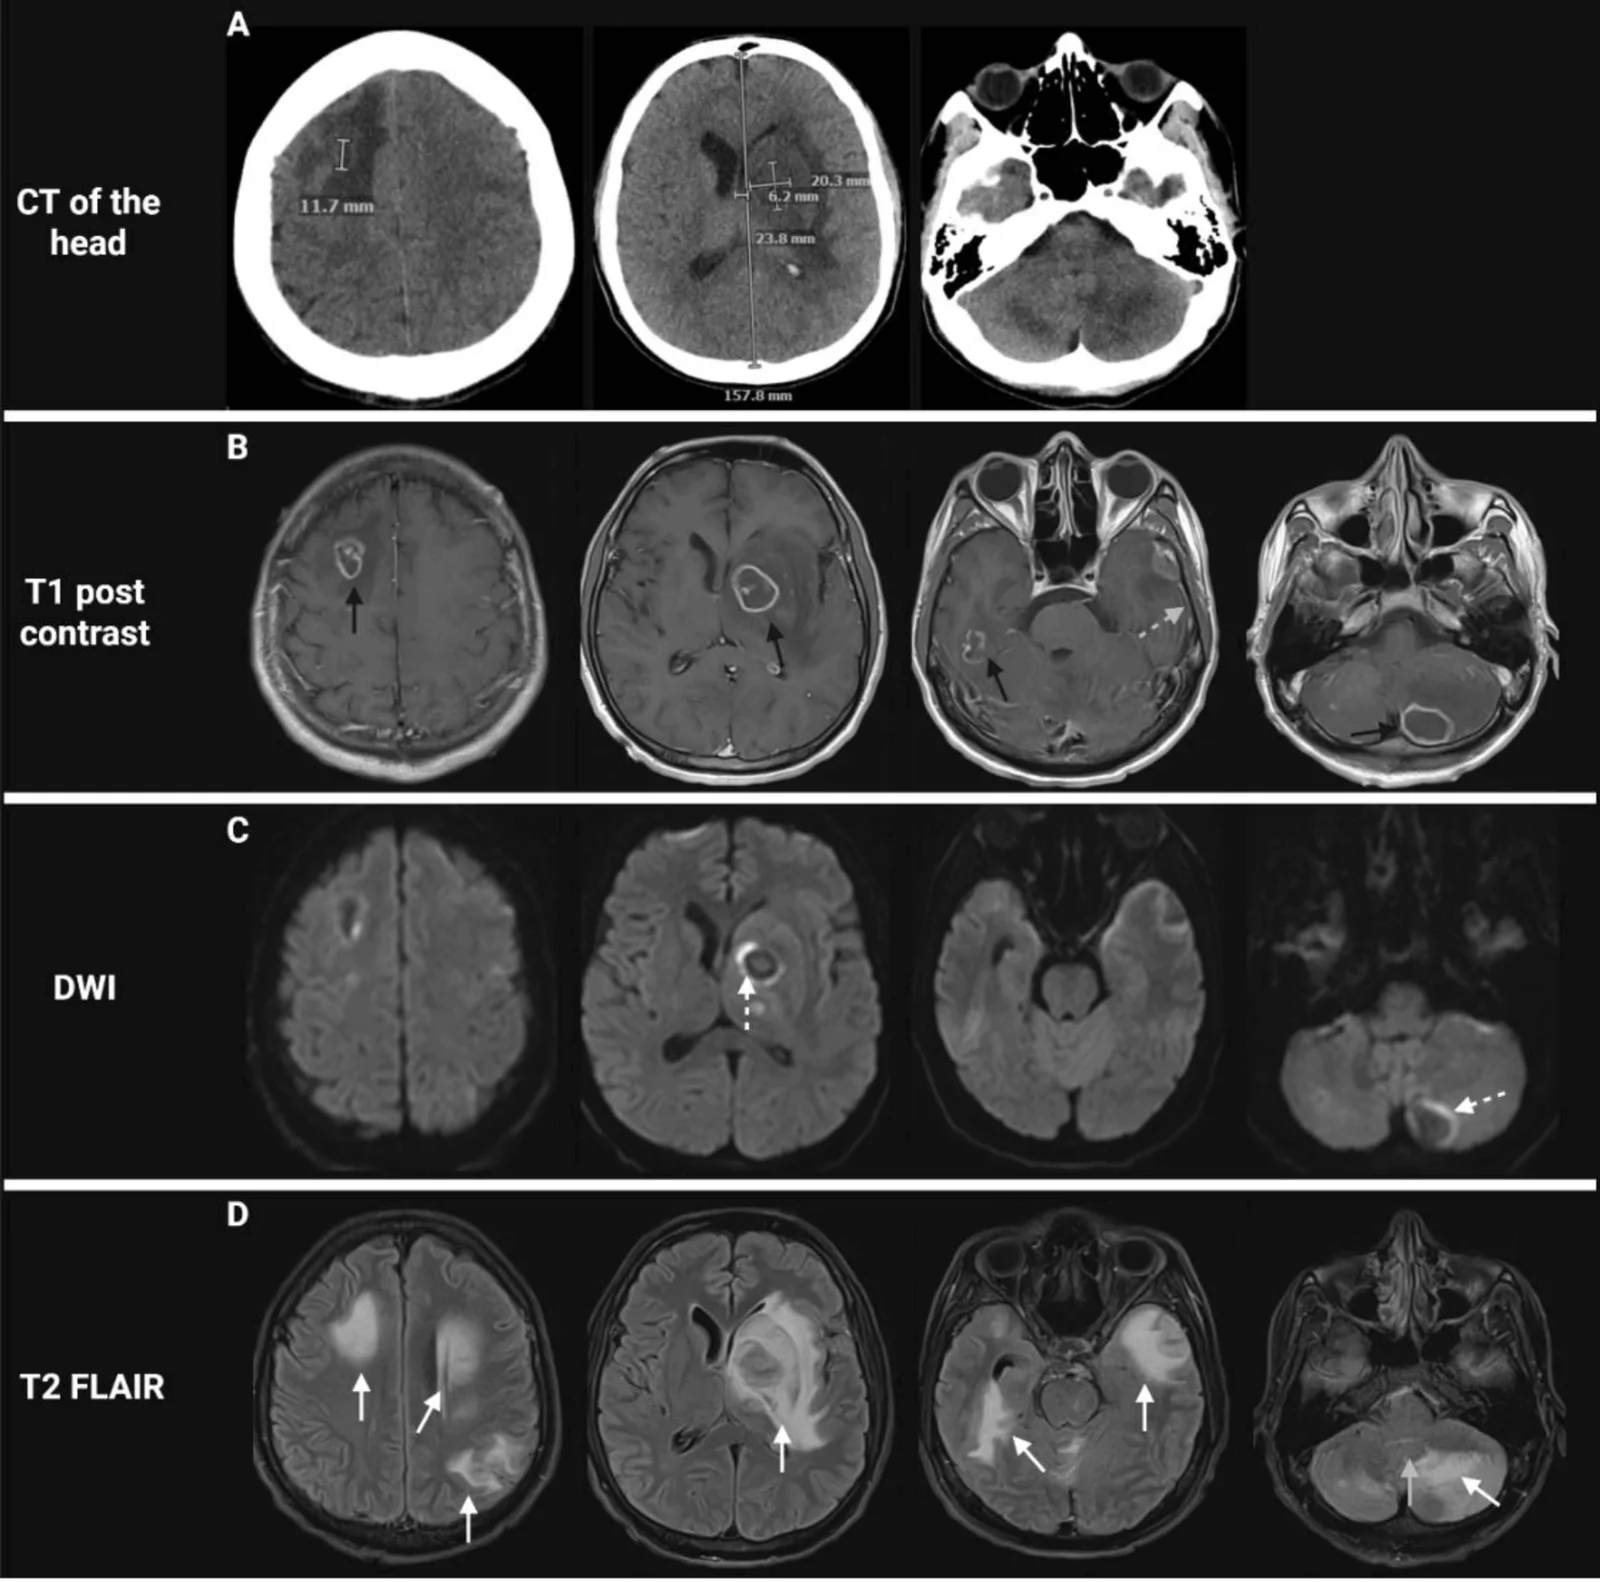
Cranial imaging showing multiple space-occupying lesions (A) CT of the head without IV contrast showing multiple supratentorial and infratentorial lesions with surrounding vasogenic edema, mass effect causing 6.2 mm midline shift, and fourth ventricular compression. (B-D) MRI of the brain with and without IV contrast demonstrated multiple ring-enhancing ((B) black arrows), peripherally diffusion-restricting ((C) white dashed arrows) supratentorial and infratentorial lesions with surrounding vasogenic edema ((D) white arrows) and mass effect on the fourth ventricle ((D) grey arrow). Some of these lesions were noted to have associated leptomeningeal enhancement ((B) grey dashed arrow). CT: computed tomography; MRI: magnetic resonance imaging; DWI: diffusion-weighted imaging; FLAIR: fluid-attenuated inversion recovery
